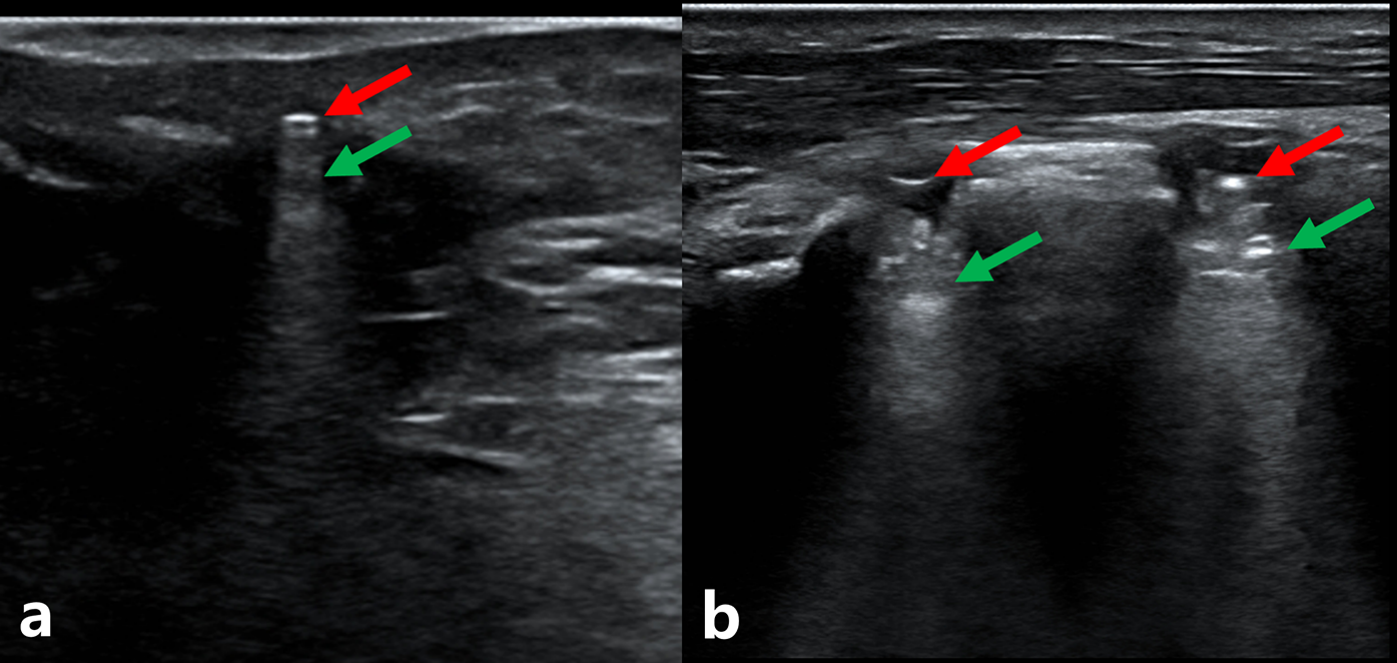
Displays the ultrasound images of a 15-year-old boy with three cannulated screws in his right tibia. (a) shows one of the screws. (b) shows the other two screws in one scan. The red arrow shows that the strong echo produced by the ultrasonic hits the screw tail. The green arrow refers to the acoustic shadow below the screw tail.
